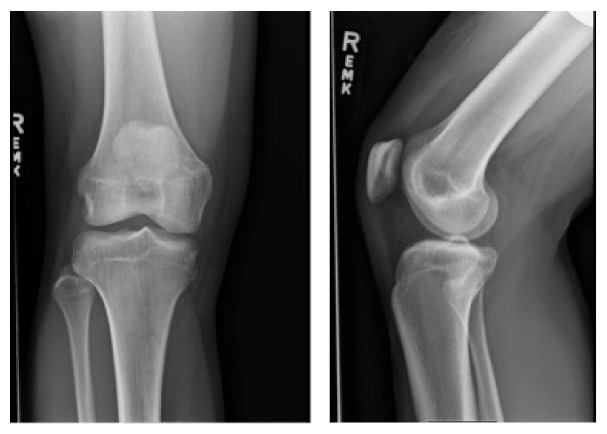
AP and lateral X-ray views of the right “unaffected” knee.
